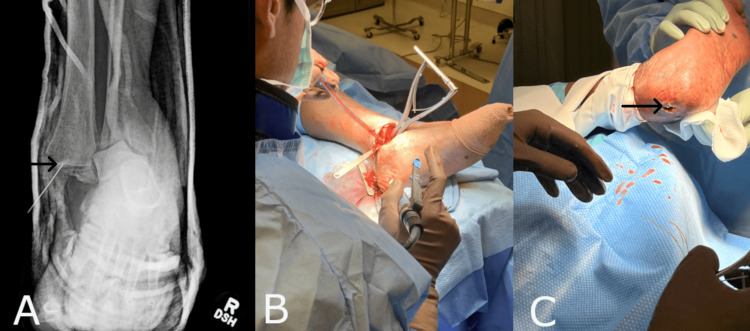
(A) Radiographic image of the ankle fracture. (B) Initial staged intervention and tibio-talo-calcaneal fusion procedure. (C) Heel wound during the I&D procedure I&D: Incision and drainage
